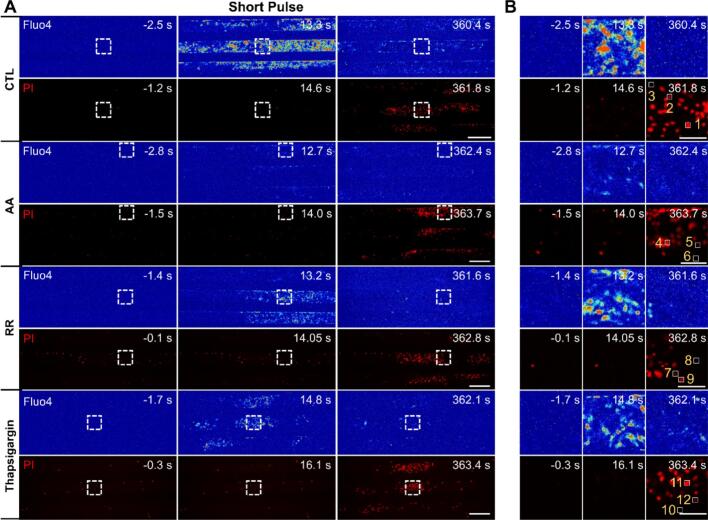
Mechanistic study for cellular Ca^2+^ response induced by 10 s short pulse ultrasound stimulation at 0.5 MPa acoustic pressure at a flow rate of 75 ul/min. (A) Image sequences of induced Ca^2+^ signaling (Fluo-4, pseudocolor) and PI uptake (red) for control groups without inhibitors, treated by ascorbic acid (AA), Ruthenium Red (RR) and Thapsigargin. Ultrasound is on from 0 to 10 s. The scale bars denote 400 µm. (B) Enlarged views of the dashed boxes in panel (A) in corresponding rows. The scale bars denote 100 µm.
